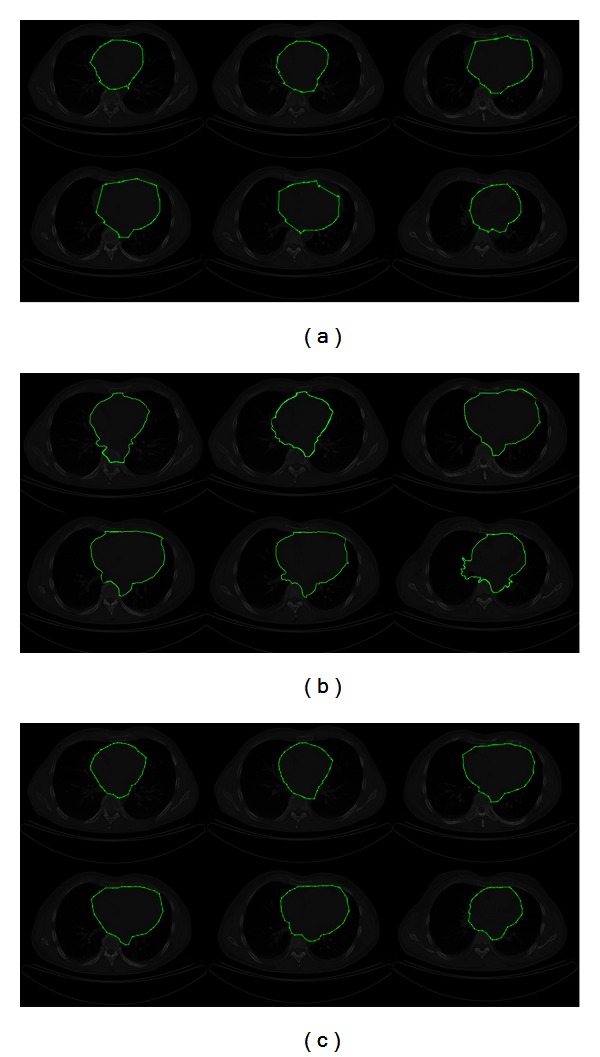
CT images (human heart segmentation): (a) results of traditional ACM, (b) results of graph cut method, and (c) results of MACPSO implementation.
